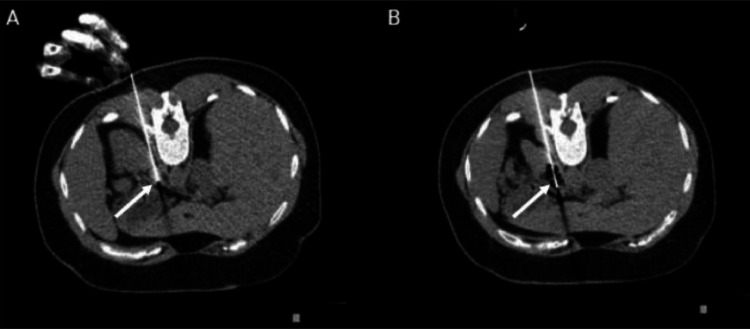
CT fluoroscopy-guided RF ablation. (A) Accurate needle placement under CT fluoroscopic guidance. (B) Conclusion of the ablation process is evidenced by gas accumulation around the needle's tip (arrow).
